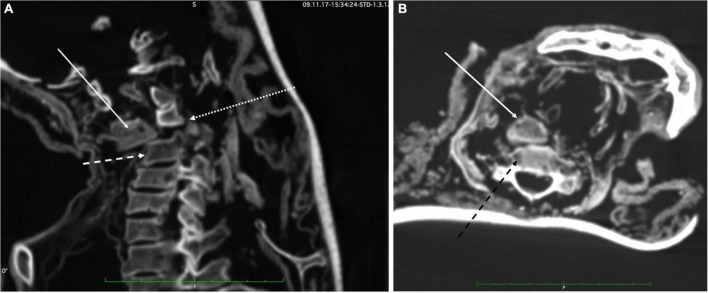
(A) Sagittal CT-scans through the cervical spine: There is a significant dislocation (small dotted arrow) between C3 (arrow) and C4 (dotted arrow) that may have resulted from a massive forward flexion. (B) The axial scan confirms the dislocation along with the surrounding intact soft tissue (white arrow = body C3; dark doted arrow = body C4).
